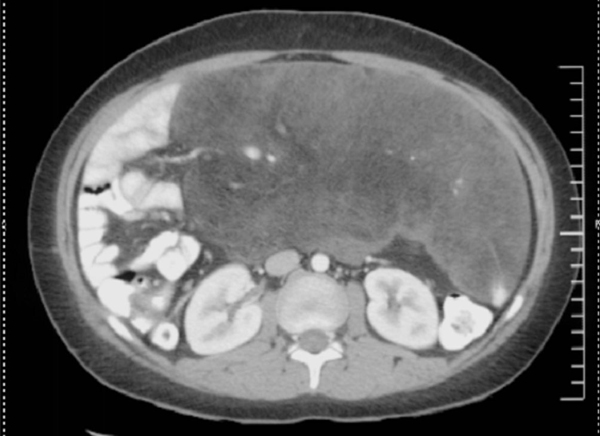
A computed tomography of a large intra-abdominal mass is seen with displacement of the surrounding structures.
